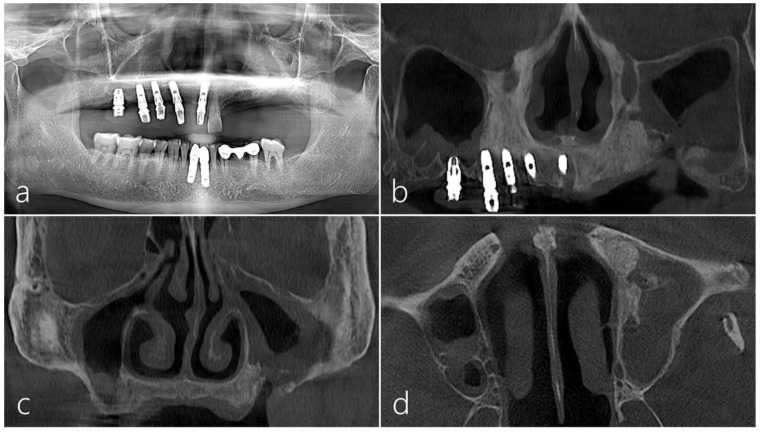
Case 1: (a) The preoperative panoramic radiograph showed a bone deficiency on the left maxillary posterior region. (b) The preoperative coronal cone-beam computed tomography (CBCT) image revealed a substantial loss in grafted bone after previous maxillary sinus augmentation. (c) On the coronal CBCT image, the mucosa of the left maxillary sinus was significantly thickened, and the natural ostium was observed to be closed. (d) The preoperative axial CBCT image showed remnants of augmented bone grafts in the left maxillary sinus.
